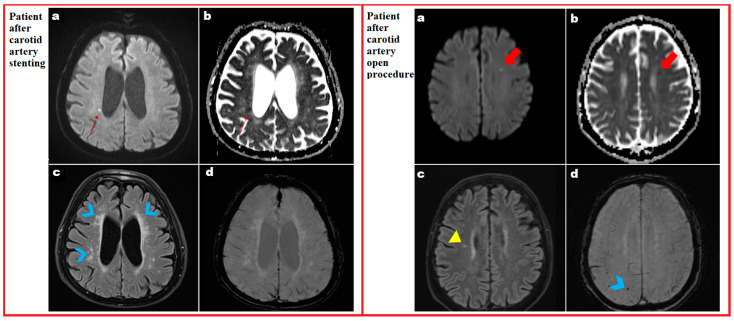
MR images of a CAS and a CEA patient. (Left side), Panel a: Right periventricular new ischemic focus with high signal intensity on DWI trace image after CAS (red arrow). Panel b: Corresponding low signal intensity on ADC map (red arrow). Panel c: Periventricular (and subcortical) FLAIR hyperintensities on both sides suggestive of severe chronic small vessel disease (blue arrows). Panel d: No signal void on SWI suggestive of a hemorrhagic component, if present. (Right side), Panel a: Left frontal periventricular new ischemic focus with high signal intensity on DWI trace image following CEA (red arrow). Panel b: Corresponding low signal intensity on ADC map (red arrow). Panel c: Mild small vessel disease presenting as FLAIR hyperintensities (yellow arrow). Panel d: Right parietal chronic micro-hemorrhage with SWI signal void (blue arrow).
